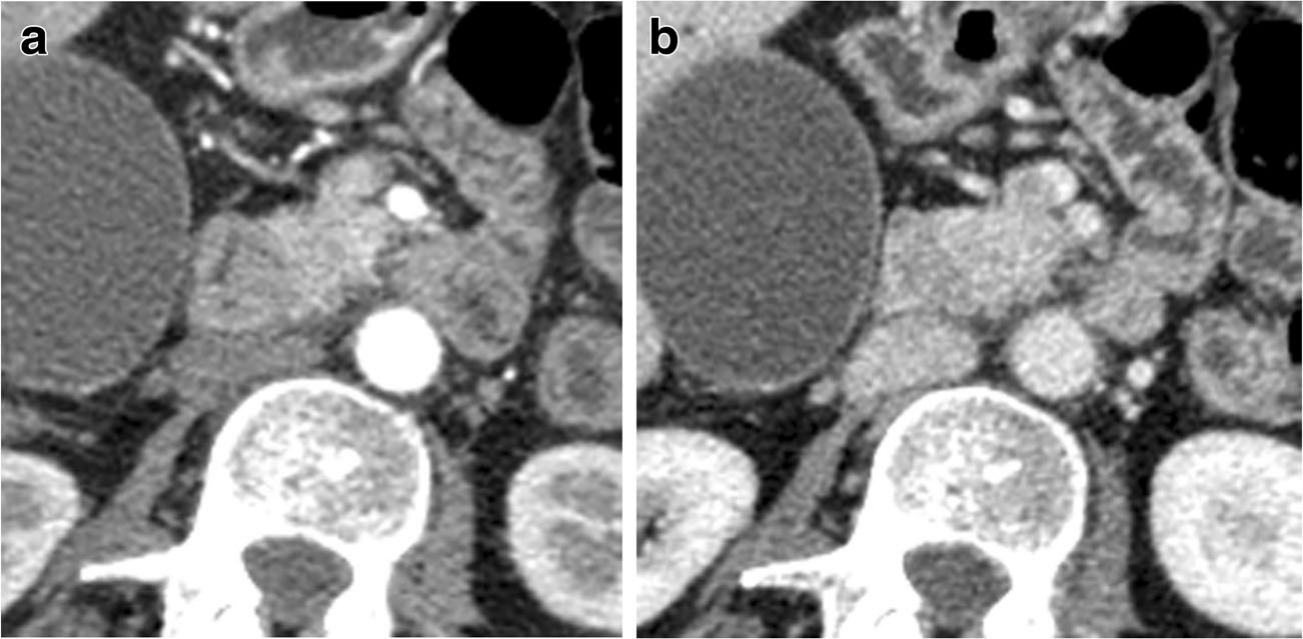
Non-functioning NEN. CT examination: small hypervascular pancreatic head mass irregular in shape invading the superior mesenteric vein with a small neoplastic thrombus studied in the pancreatic (a) and venous (b) phases
